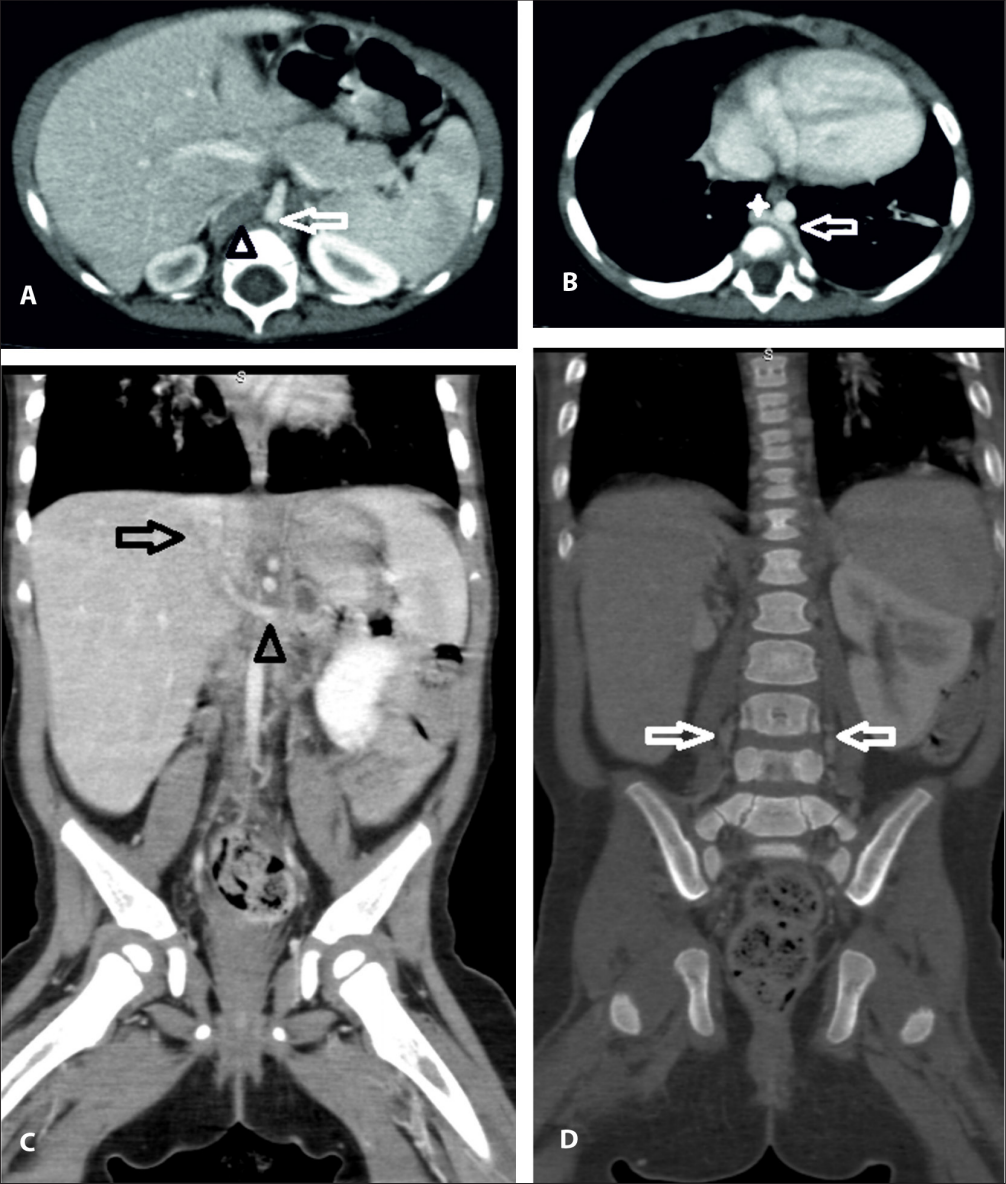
Contrast-enhanced computed tomography, showing in the axial plane (A) hemiazygous vein (arrow) draining into a continuous azygous vein (asterix) and (B) absence of opacification of the inferior vena cava (arrowhead) adjacent to aorta (arrow). Coronal reformation images showed (C) dilated ascending lumbar veins (arrows) and (D) the drainage of the left renal vein (arrowhead) into the intrahepatic segment of IVC (arrow).
